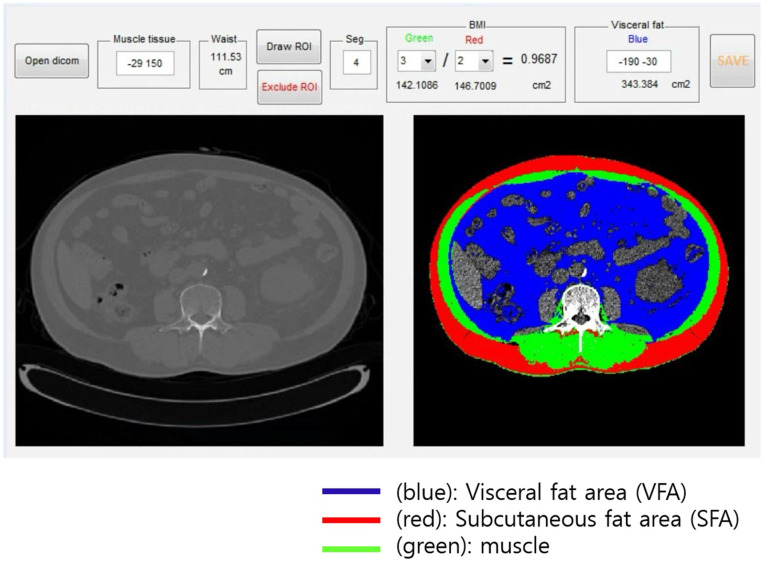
Semiquantitative body mass index and subcutaneous fat computed tomography analysis.
